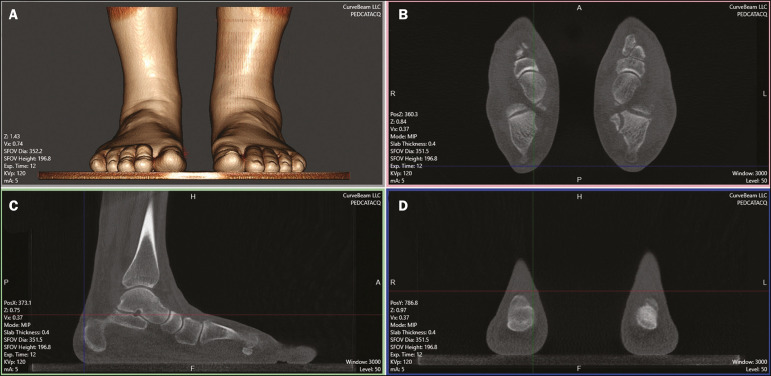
3D reconstruction (A) and multiplanar reconstructions (B,C,D), achieved with CubeVue software (CurveBeam LLC, Hatfield, PA, USA) after volumetric acquisition with weight-bearing CBCT.
